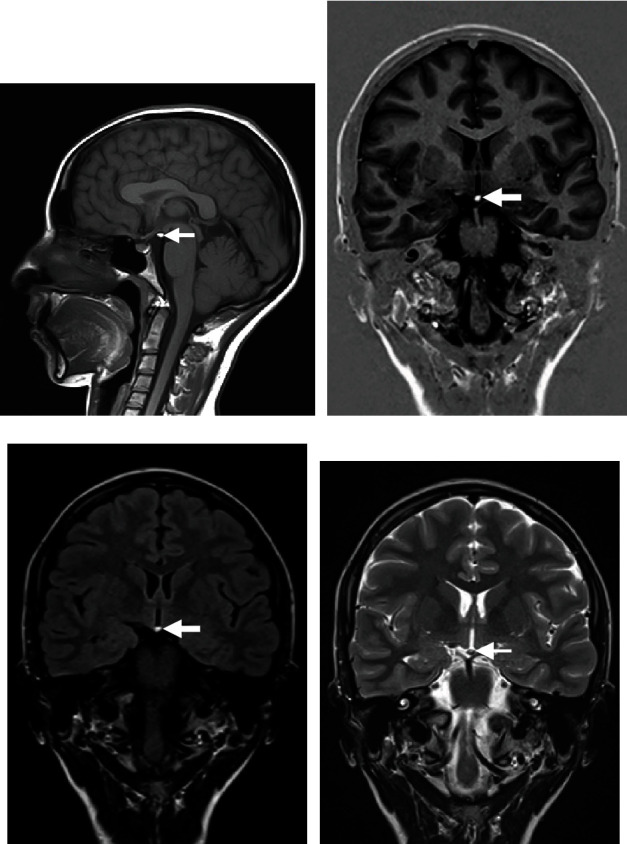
(a) Sagittal T1-weighted MRI. (b) Coronal T1-weighted gradient MRI (FSPGR). (c) Coronal FLAIR T2-weighted MRI. (d) Coronal T2-weighted image. (a)–(d) A small hypothalamic lipoma is noted, following fat signal on all sequences.
